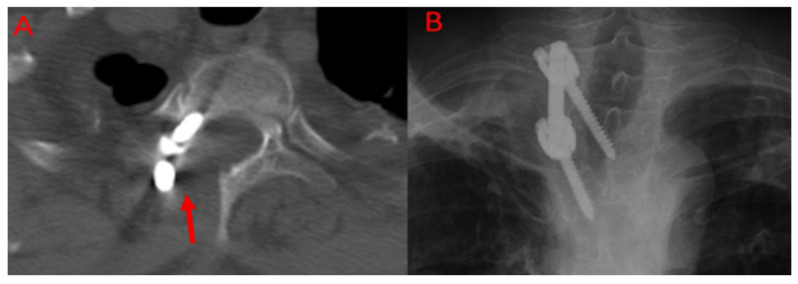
Imaging of a patient with a thoracic spinal hydatid cyst after unilateral CLDS. (A): Axial CT image highlighting the laminectomy site and stabilisation hardware used in the unilateral CLDS procedure (the arrow indicates the surgical instruments and laminectomy site). (B): Anteroposterior (AP) X-ray of the same patient, demonstrating the posterior instrumentation.
